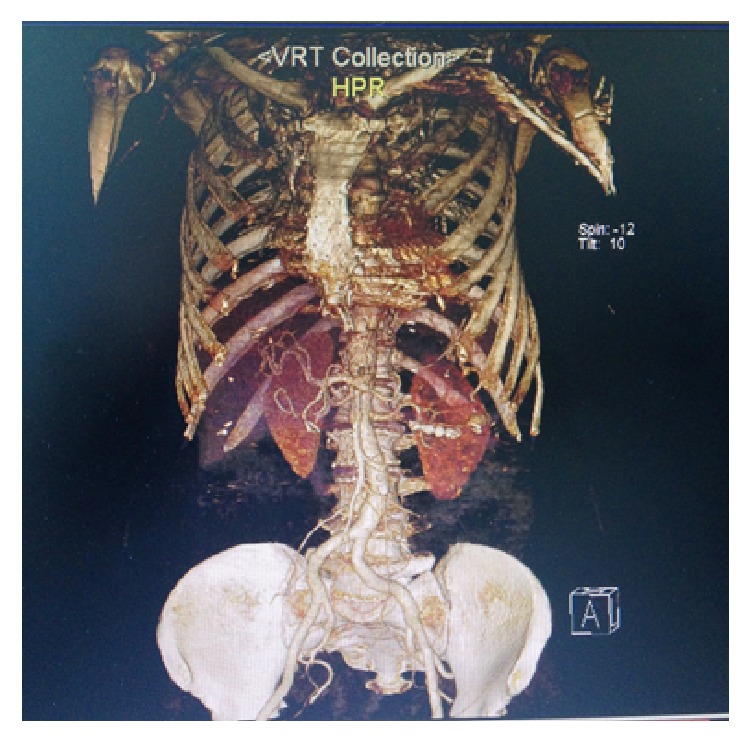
After three months, CT angiography showed thrombosed aneurysm sack and normal spleen.
